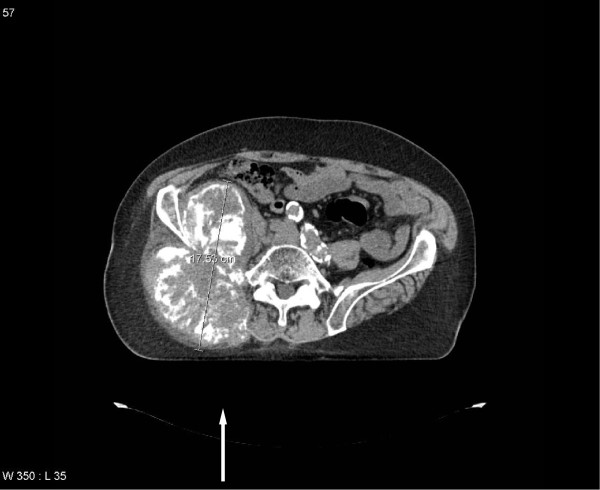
Computed tomography scan in a patient with adenocarcinoma of the lung (no EGFR mutation) and bone pain (bone lesion causing weakness of the right lower limb, initial manifestation of this lung malignancy, treatment-naïve), massive extra-osseous extension present: treated with 3 Gy x10.
